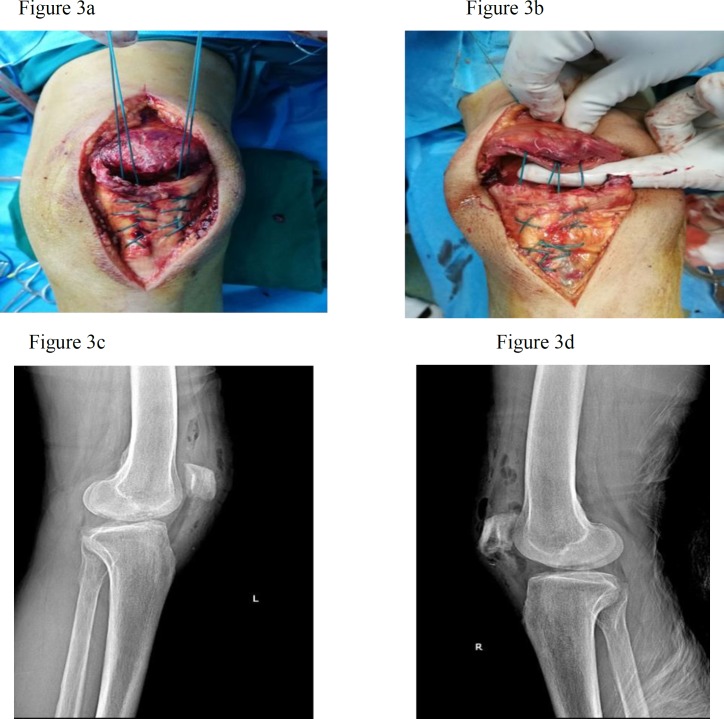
Technique of patellar tendon repair with bone tunnel, (a) and (b). Post-operative lateral radiography of Lt and Rt knees showing patella in normal position, (c) and (d)
